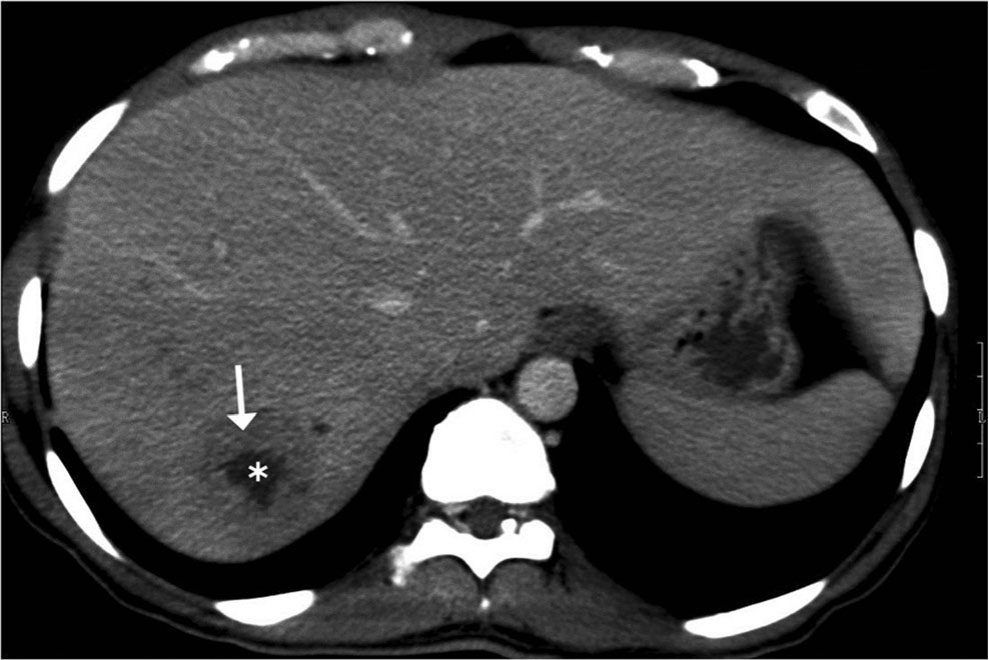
A case of nodular isolated hepatic tuberculosis. Axial contrast-enhanced CT shows a well-defined lesion in the right lobe with thick enhancing rim (*arrow*) and central area of necrosis (*asterisk*)
